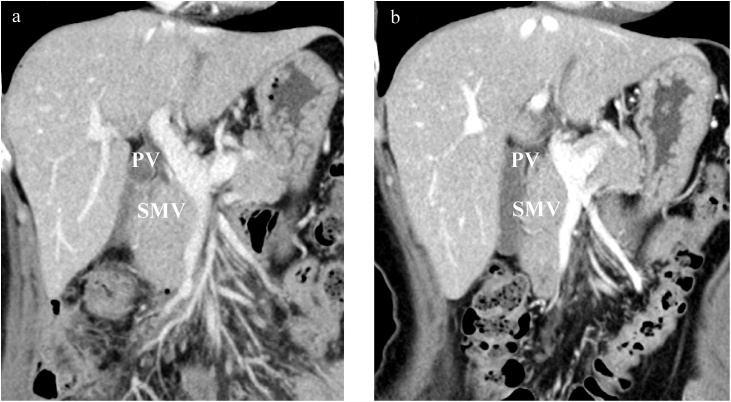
Postoperative findings. (a) The patency of the reconstructed vein was sustained and remnant tumor thrombosis was not detected on the seventh postoperative day. (b) No recurrence has been confirmed at 17 months follow-up CT imaging.
